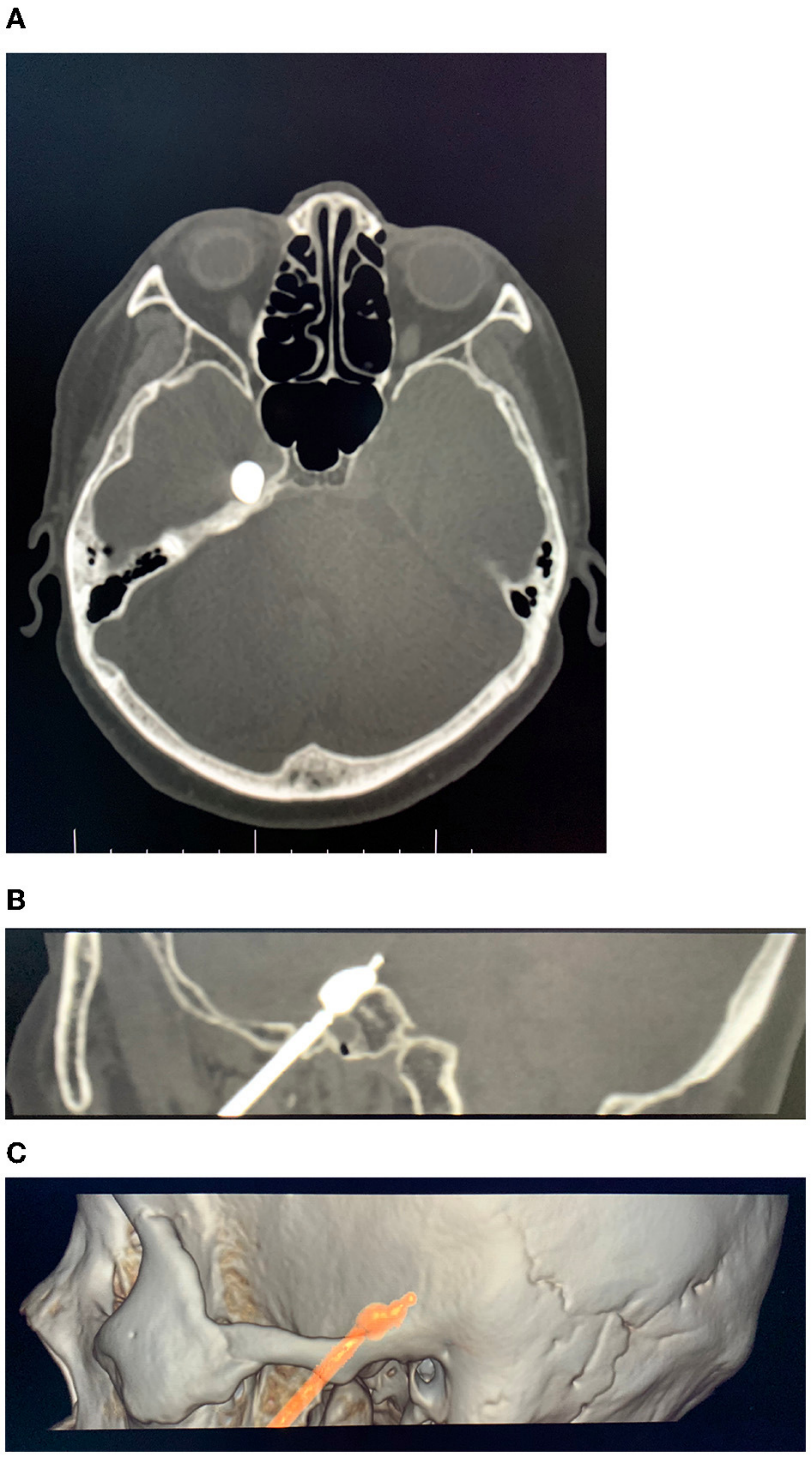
Axial (A) and sagittal (B) computed tomography (CT) reconstruction images showing the balloon shape and position were correct (the white arrow points to the balloon). (C) Entry point and target direction.
